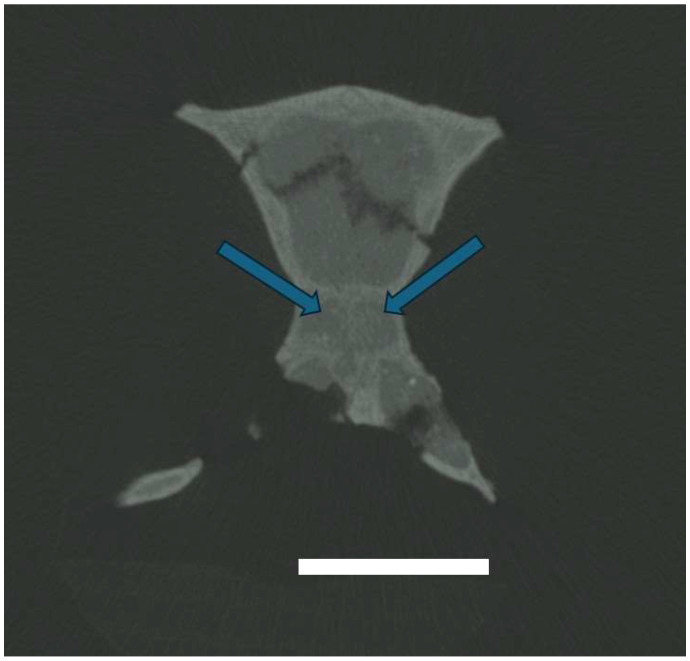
CT scans (slide 208) show the olfactory recess of *Mioeuoticus shipmani* (KNM-RU 2052; holotype), with blue arrows highlighting the change in resolution that suggests the presence of a fourth pair of ethmoturbinals. Scale bar is 5 mm.
